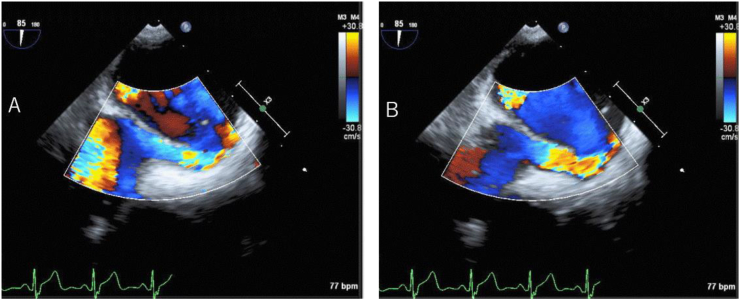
Transesophageal echocardiography (TEE). During TEE, the posture was changed from the supine position (A) to the sitting position (B).
